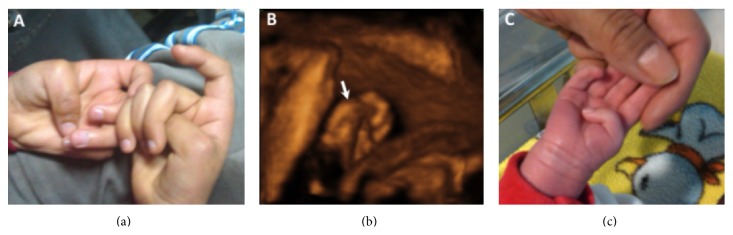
Case 1. (a) Picture of the hands in the index case shows bilateral adducted thumbs. (b) Surface-rendered three-dimensional ultrasound view shows adducted thumb in the fetus at 30 weeks (arrow). (c) Picture of the newborn infant shows adducted thumb.
